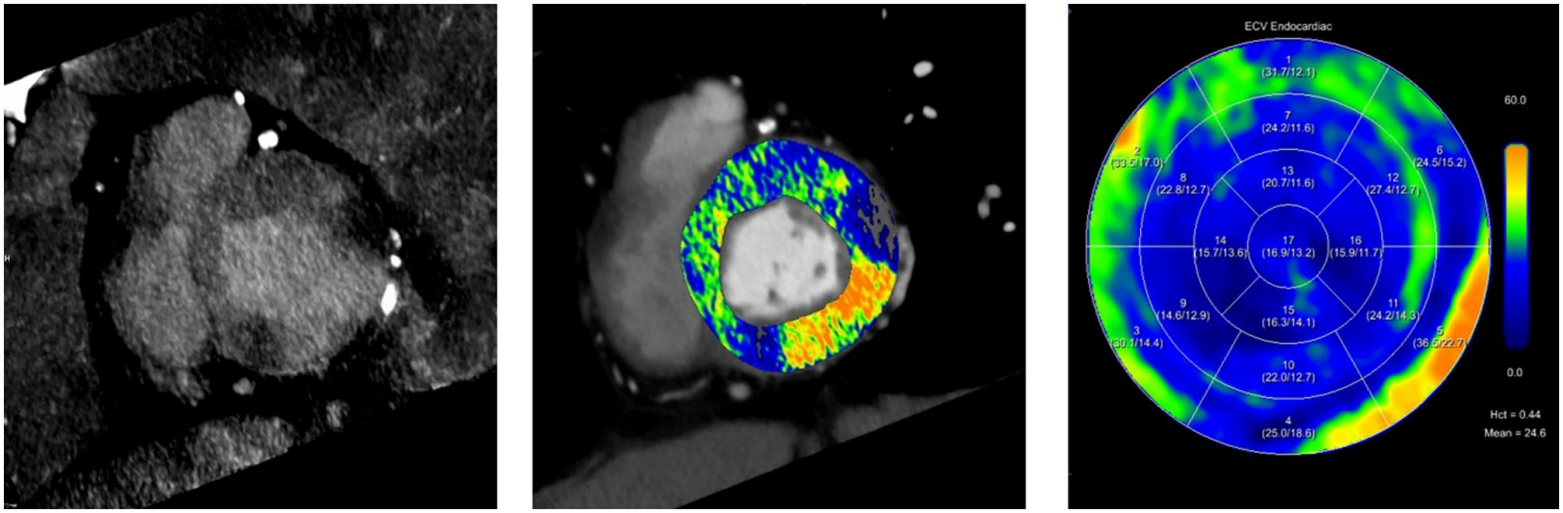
A 83-year-old male patient with severe aortic stenosis undergoing dual-source photon-counting detector CT including a late enhancement scan acquired 5 min after contrast media administration. Iodine map of the late enhancement CT scan and extracellular volume (ECV) polar maps (right) demonstrate pathological late enhancement in the basal inferolateral left ventricular wall. Elevated ECV values are shown in the corresponding territory (max ECV = 36.5 ± 22.7) in overlay (middle) and polar maps (right).
